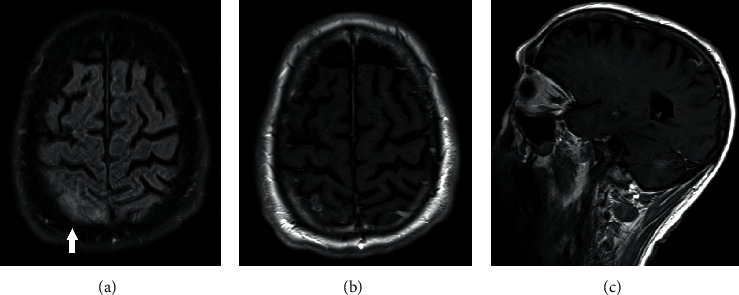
Fluid-attenuated inversion recovery images (FLAIR) showed a “dirty CSF” appearance in the right parietal region ((a), white arrow). Axial (b) and sagittal (c) T1-weighted images after the intravenous administration of gadolinium-chelate revealed a focal area of superficial contrast enhancement overlying the cerebral cortex, presumably representing a thickened pia mater.
